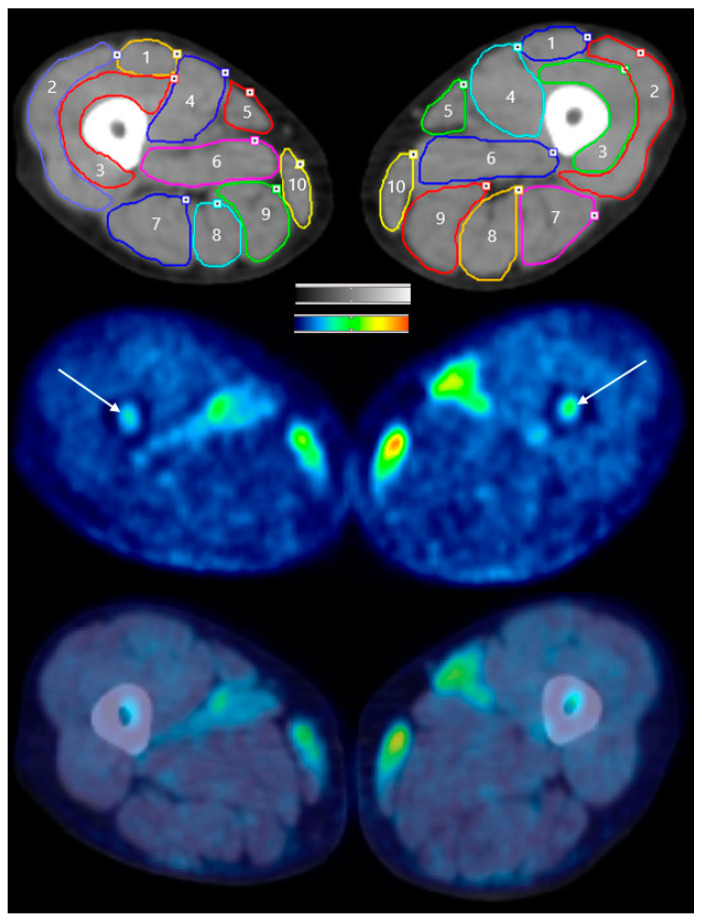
Cross-sectional images at the mid-thigh level of Subject 2 in the tDCS condition. The top image is a computed tomography (CT) scan with regions of interest (ROIs) circled to indicate the location of the skeletal muscles. 1 = Rectus Femoris, 2 = Vastus Lateralis, 3 = Vastus Intermedius, 4 = Vastus Medialis, 5 = Sartorius, 6 = Adductor Magnus (not included in the analysis), 7 = Biceps Femoris, 8 = Semitendinosus, 9 = Semimembranosus, 10 = Gracilis. The ROIs drawn on the CT image were transferred to the positron emission tomography (PET) image (middle image). The white arrows indicate bone marrow metabolism. The bottom image is of the co-registered CT and PET images. Red denotes the greatest signal intensity, followed by yellow, green, and blue.
